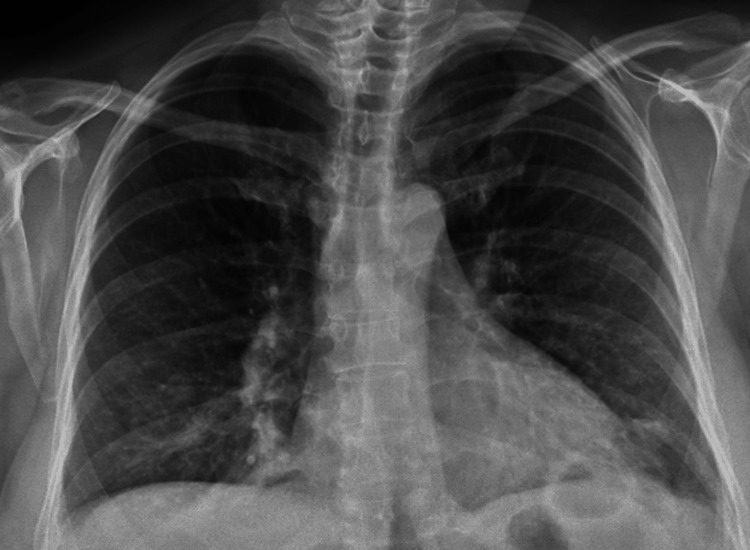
Chest X-ray on presentation, reported to have no acute lung pathology
